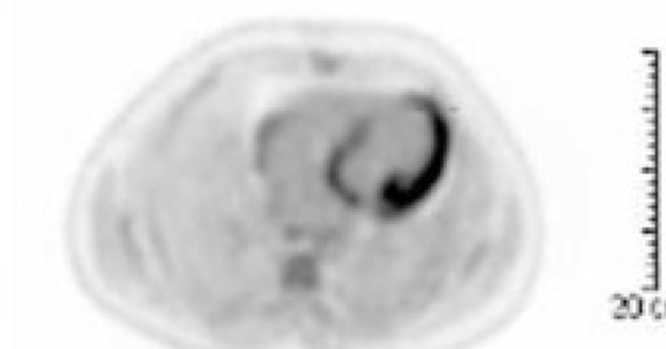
Fused Positron Emission Tomography/Computed Tomography Images Demonstrating Focal Fluorodeoxyglucose Myocardial Hypermetabolism (Maximum Standardized Uptake Value 6.6)
